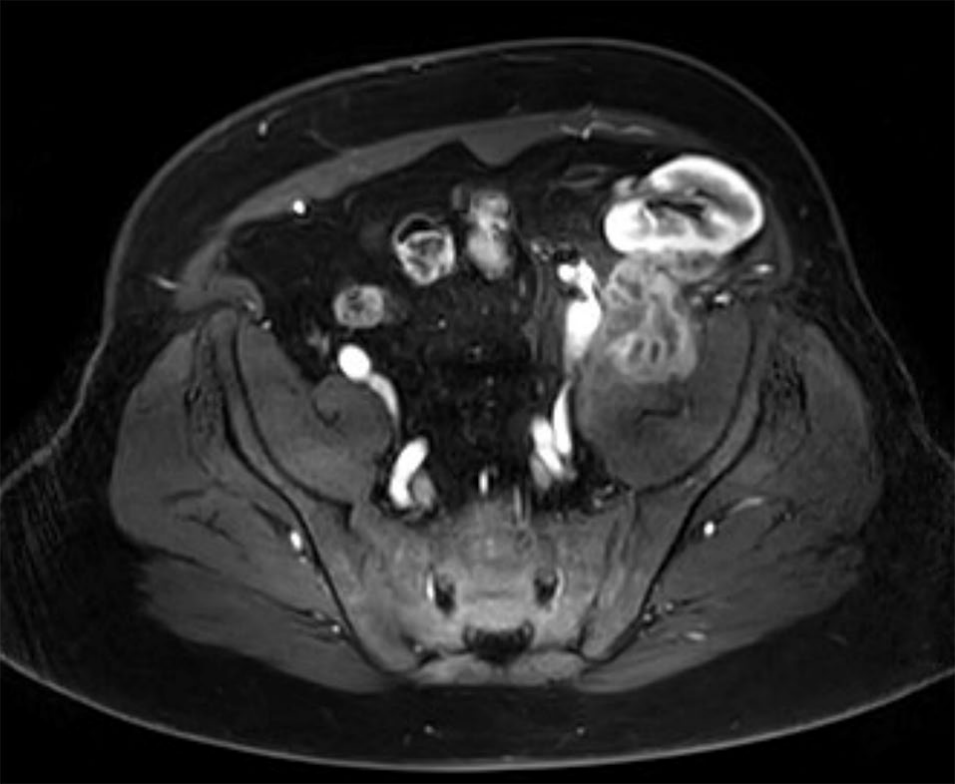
MRI of the collection following the first aspiration and drainage. A thick capsule is observed below the renal graft, compressing it on the posterior wall, initially raising suspicion of a tumoral nature
